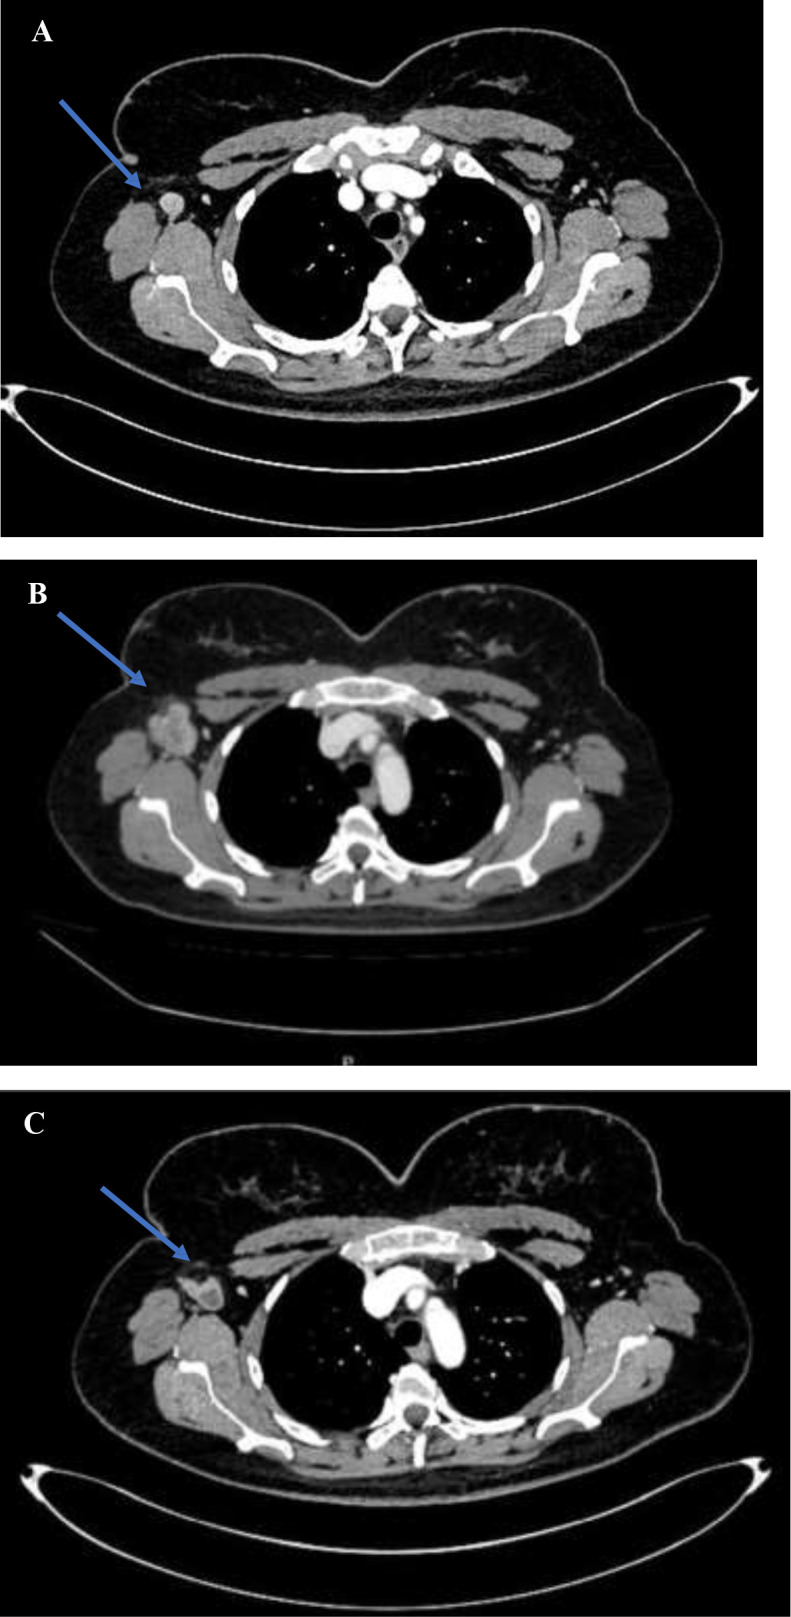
(A) CT-Scan performed in February 2025, before neoadjuvant immunotherapy. (B) CT scan in May 2025, after three cycles of neoadjuvant immunotherapy. (C) CT- Scan performed in August 2025, after three months of target therapy.
